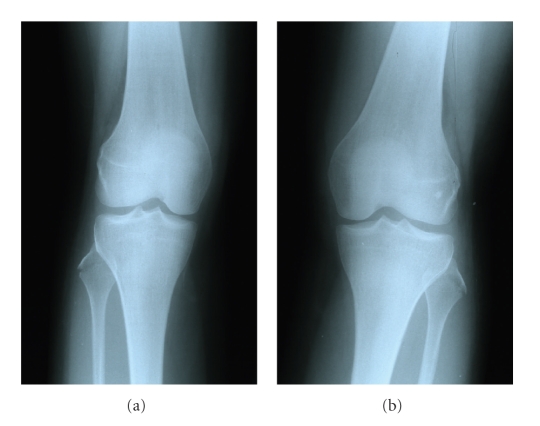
Anteroposterior radiographs of both knees immediately after trauma. Fractures of the lateral borders of both fibular heads are easily seen.
